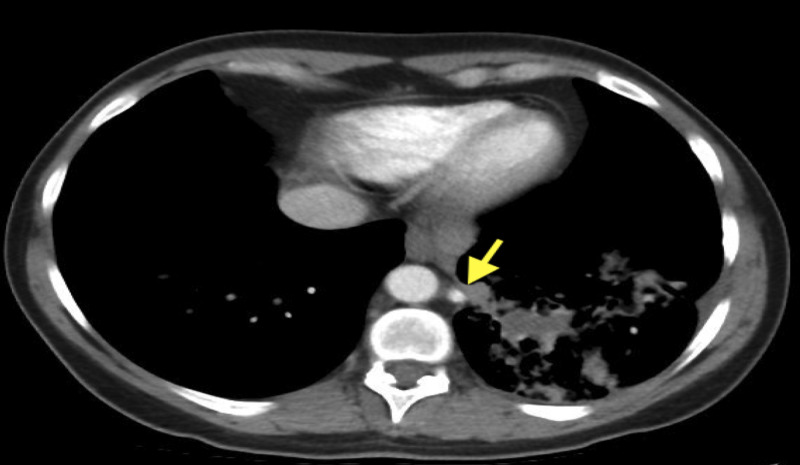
CT chest with contrast. The yellow arrow shows feeding artery entering area of consolidation.
